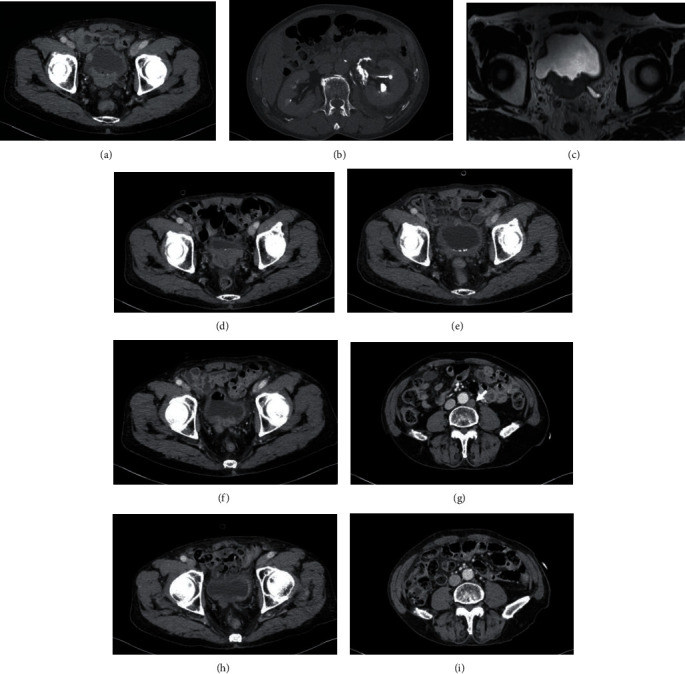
Imaging findings. (a) CT in April 2019 at diagnosis shows the extramural invasive tumor in posterior and trigonal bladder wall. (b) CT urography at diagnosis shows the leakage of contrast media because of extravasated urine. (c) MRI at diagnosis shows that the extramural invasive tumor in posterior and trigonal bladder wall is a low signal intensity on T2-weighted image. (d) CT in December 2019 at the discontinuation of pembrolizumab therapy shows growth in the primary lesion. (e) CT in June 2020 after radiotherapy shows diminished primary tumor in size. (f) CT in September 2020 three months following radiotherapy shows regrowth of the primary lesion. (g) CT in September 2020 three months following radiotherapy shows the emergency of para-aortic lymph node metastasis (arrow: para-aortic lymph node metastasis). (h) CT in February 2021 at the end of 8 doses of pembrolizumab therapy resumption shows significant reduction of the primary lesion. (i) CT in February 2021 at the end of 8 doses of pembrolizumab therapy resumption shows almost disappearance of para-aortic lymph node metastasis.
